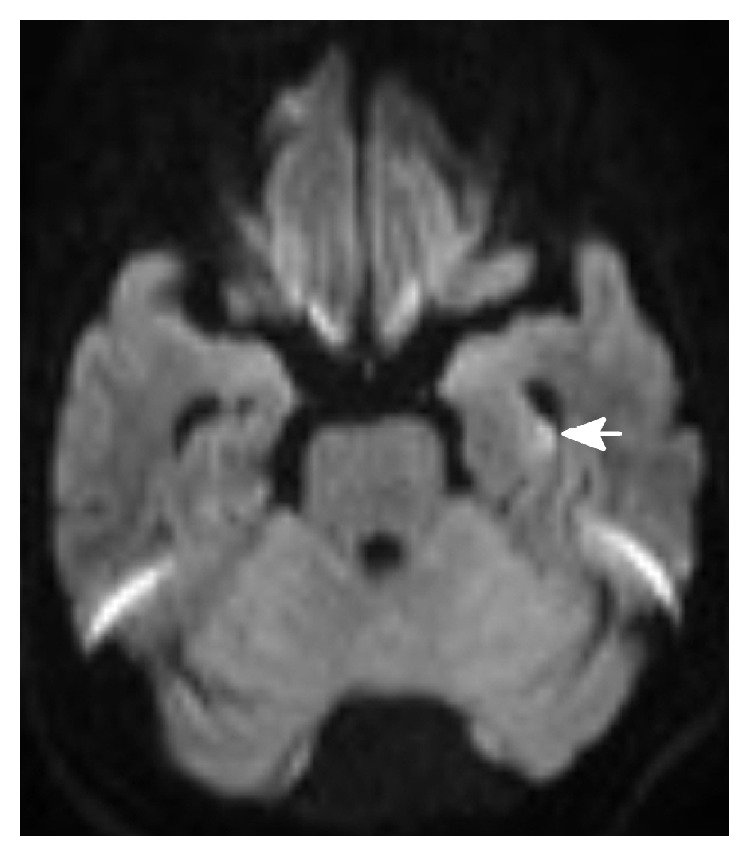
DWI of case 4 after TGA showing small acute infarctions (arrow) in left hippocampus.
